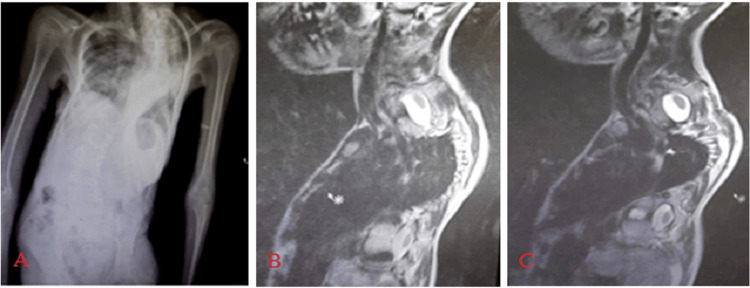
An 11-year-old male AIS patient with Dural ectasia seen at thoracic region with possible foraminal cyst seen left side of upper thoracic region measures about 15 mm on preoperative MRI. A: Chest X-ray showing scoliosis, B and C: sagittal MRI showing left lower cervical dural ectasia seen in the left upper thoracic region measuring 15 mm
